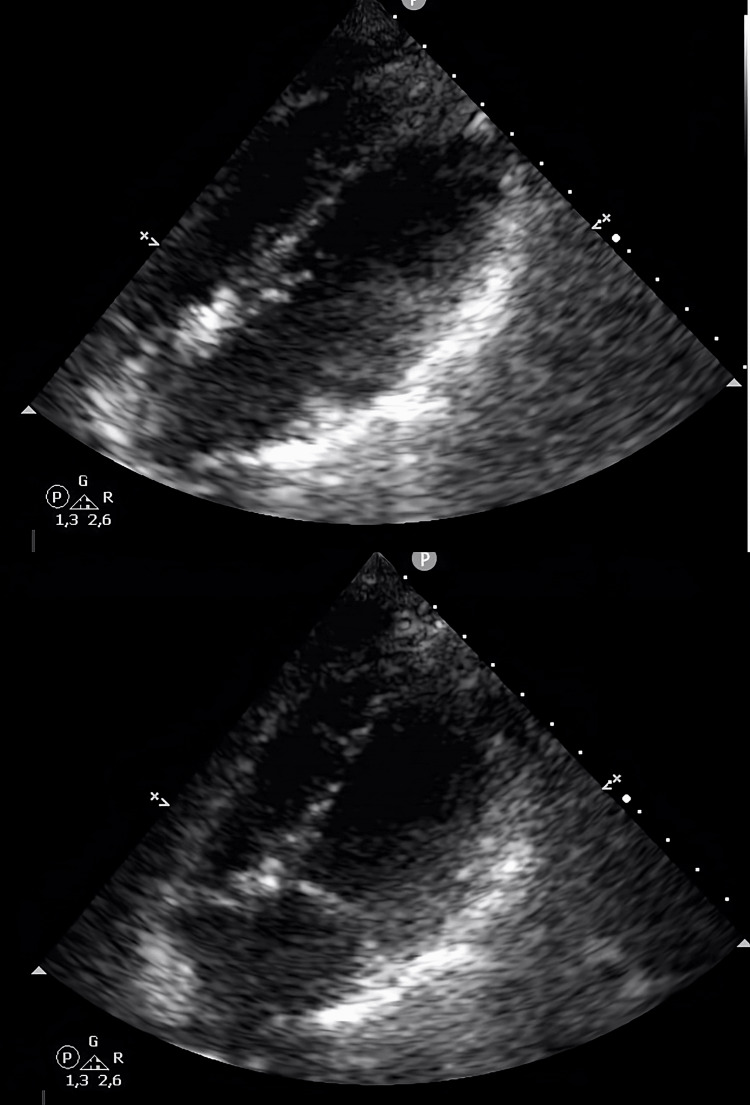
The second control transthoracic echocardiography showed normal ventricular kinetics.
